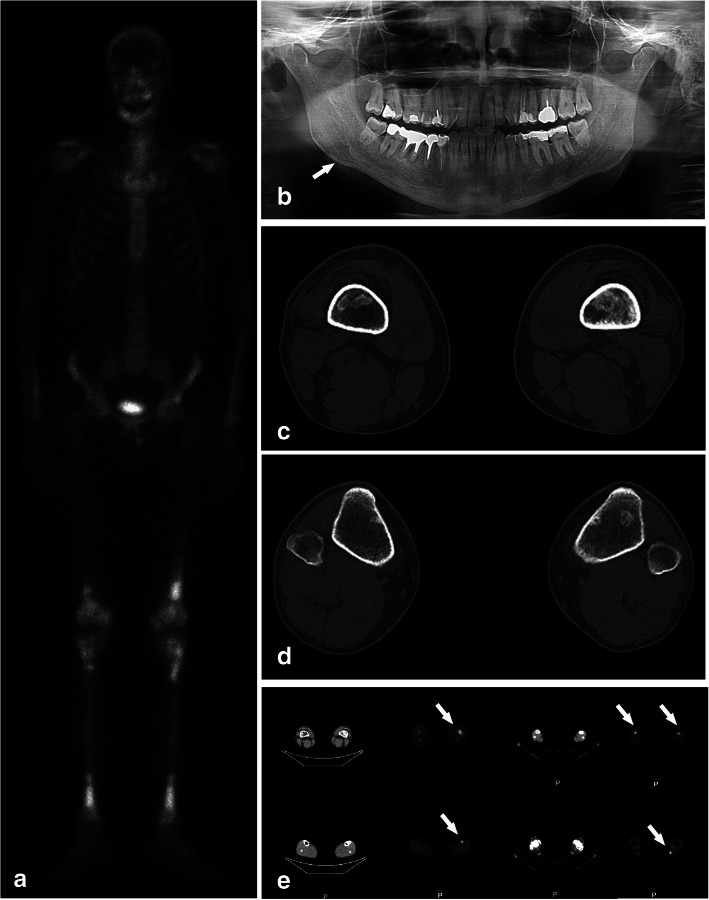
Bone scans findings suggestive of ECD. ^99^Tc-MDP bone scintigraphy (a) demonstrating multiple areas of abnormally increased radiotracer uptake including the distal femurs and symmetric uptake in the tibiae, with additional areas of weak uptake in the ulnae, radii, right mandible, left maxilla, thoracic vertebrae. Orthopantomogram (b; panorex) demonstrating lucency (arrow) in the right mandible. CT scans of distal portions of the thighs (c) and legs (d) showing medullary areas of osteosclerosis without cortical erosions in the femurs and tibiae. Similarly, PET (e) showing increased FDG uptake in multiple skeletal locations. As in the case of the bone scan, the symmetric uptake in the lower extremities (arrows) is strongly suggestive of ECD
